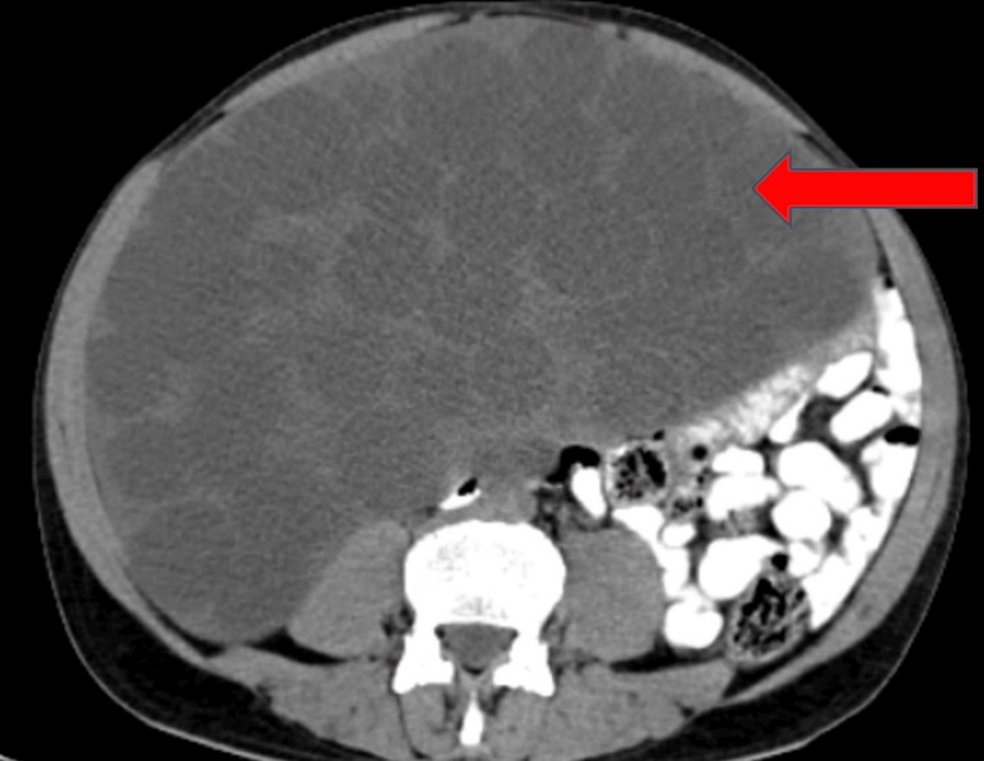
A CT of the abdomen showing multiple hydatid cysts in the peritoneum (red arrow)
